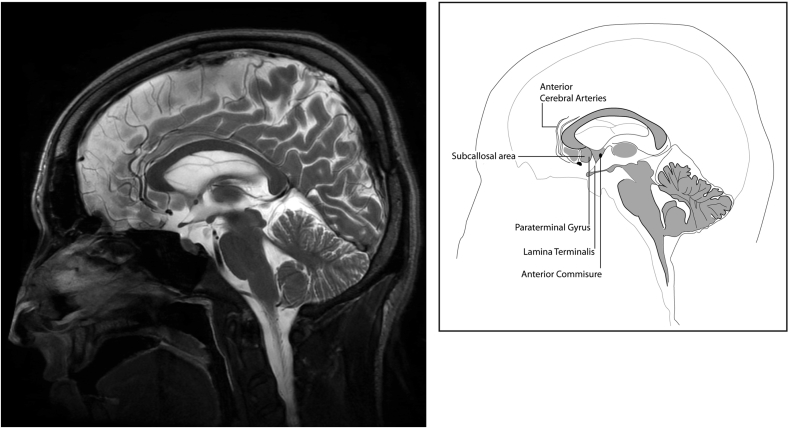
Sagittal T2-weighted image of an adult brain demonstrating the anatomy of the septal area.
